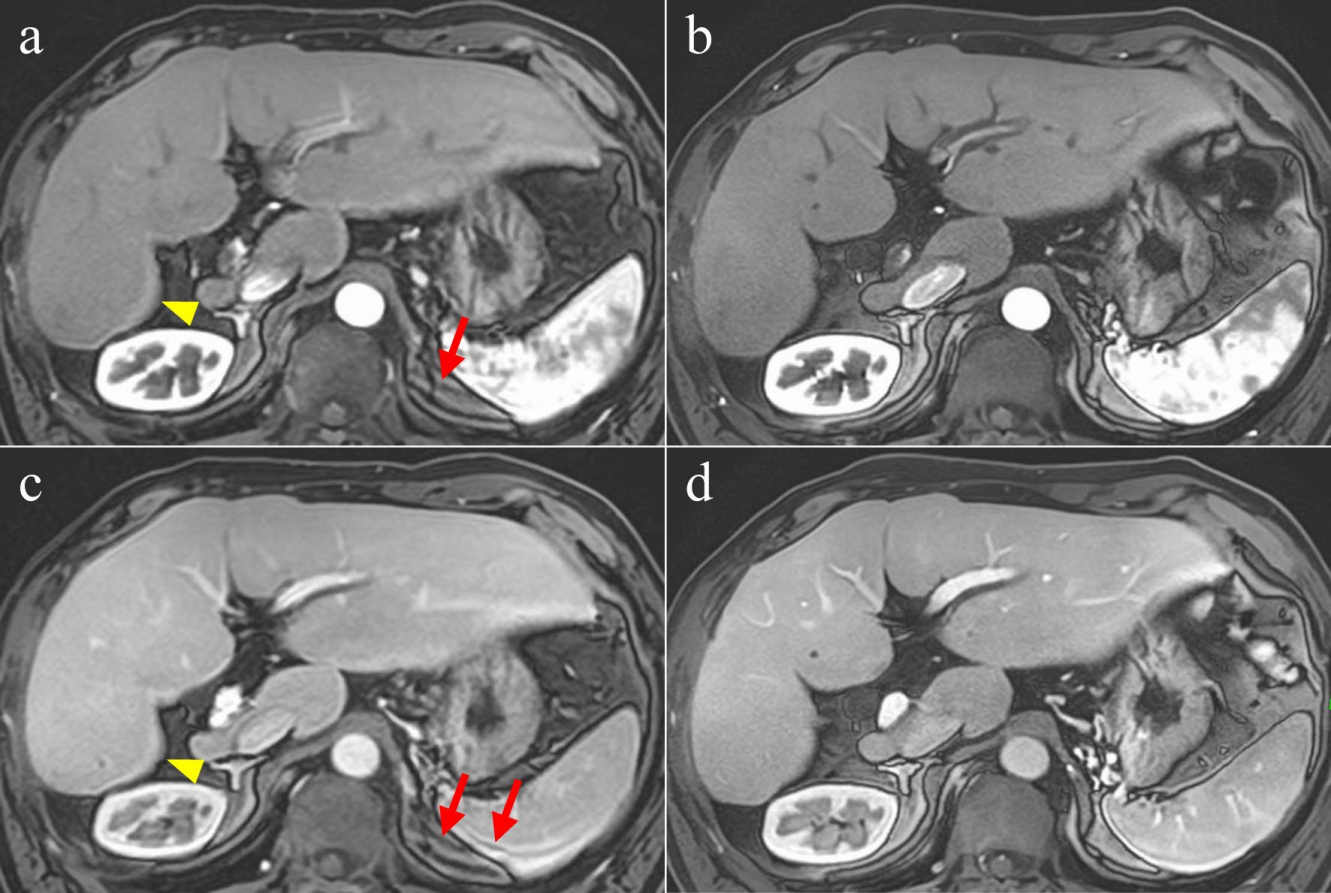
Multiphasic contrast-enhanced three-dimensional (3D) dynamic MR imaging of the abdomen during a breath holding. a CS with AiCE in arterial phase (AP), b a mFast 3D wheel using AiCE in AP. c CS with AiCE in portal venous phase (PVP), and d a mFast 3D wheel using AiCE in PVP. Visibility of intrahepatic vessels and overall image quality in the mFast 3D wheel using AiCE (b, d) were significantly better than those in CS with AiCE (a, c) in both AP and PVP images. Artifacts due to the motion (arrows) and edge enhancement (arrowheads) were observed in CS with AiCE (a, c). *CS* compressed sensing, *AiCE* advanced intelligent clear-IQ engine, *mFast* 3*D wheel* modified fast 3D mode wheel with sequential data filling
